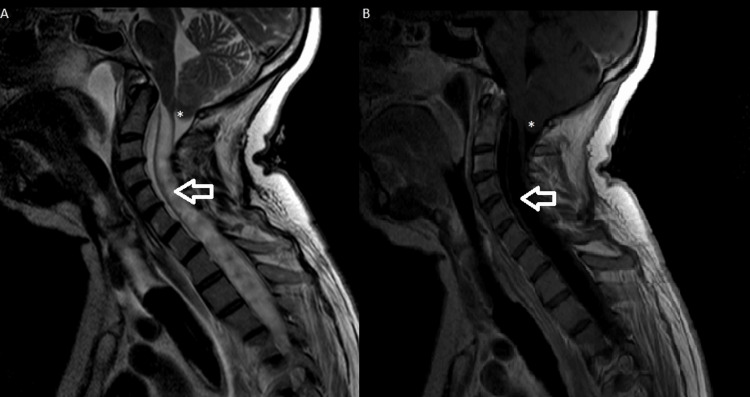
Magnetic resonance imaging, T2-weighted (A) and T1-weighted (B), performed at diagnosis before surgery. Cerebellar tonsillar herniation at the foramen magnum, significantly compressing the bulbo-medullary junction (white asterisk), with an extensive syringomyelic cavity extending through the cervical and dorsal spine (white arrow).
